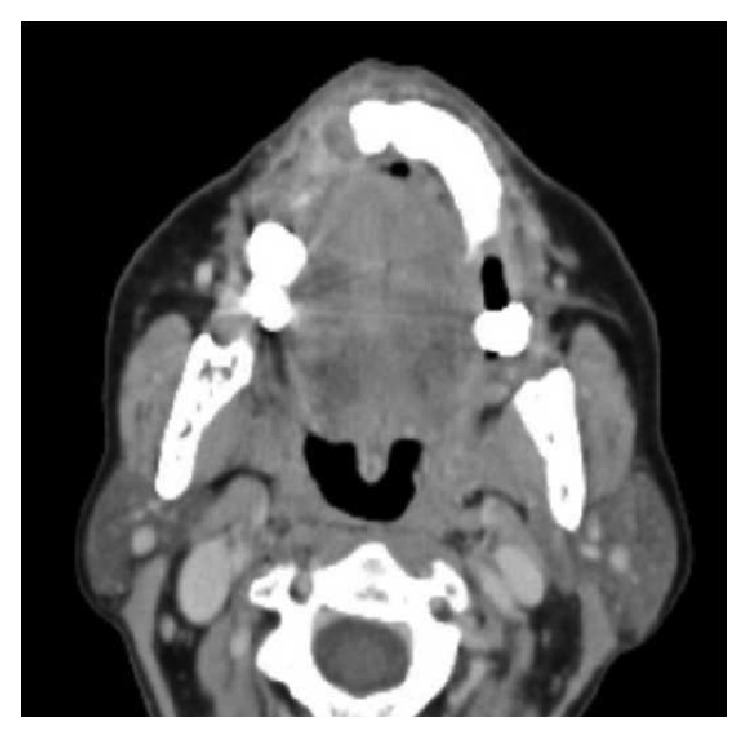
Preoperative axial CT scans with contrast showing left upper alveolar crest lesion.
